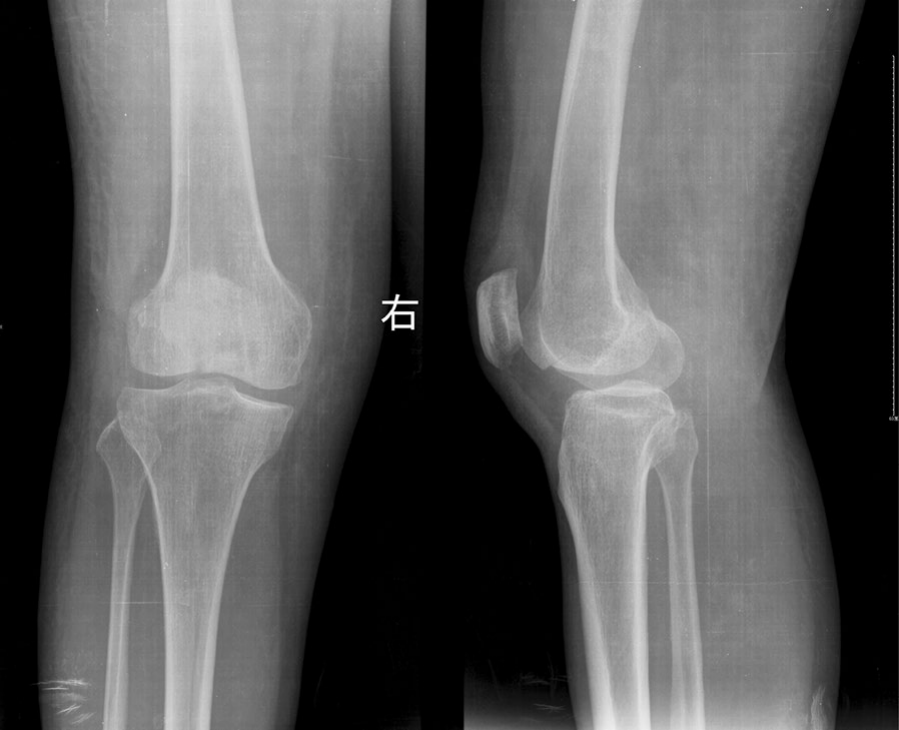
Anteroposterior and lateral radiographs show Hoffa fracture (type III) of the lateral femoral condyle
